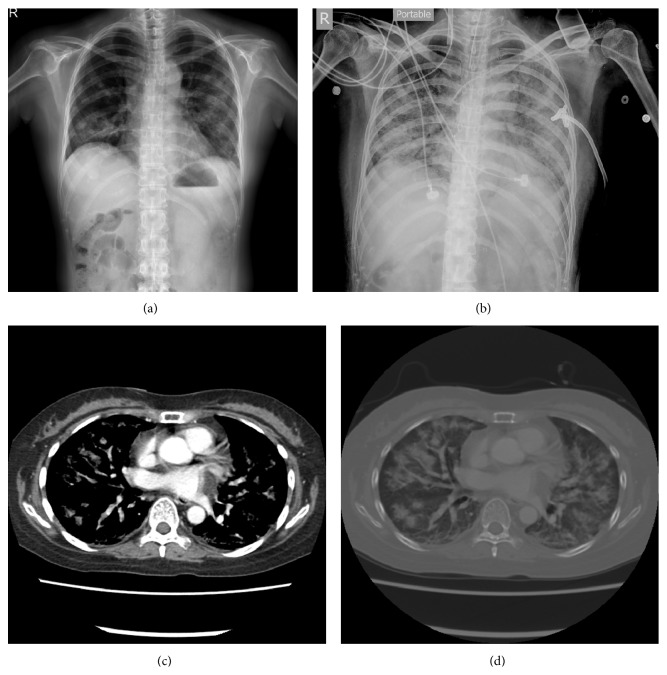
(a) Admission anteroposterior chest radiography shows multilobar solitary nodules and consolidation. (b) Anteroposterior chest radiography on hospital day 10 shows diffuse consolidation of bilateral lungs and pneumothorax with subcutaneous emphysema in soft tissues. (c) Chest CT scan (contrast) shows diffuse irregular rim of consolidation and multiple solitary nodules of bilateral lungs. (d) Chest CT scan (lung window) shows diffuse consolidation with multiple air lucencies and solitary nodules of bilateral lungs.
